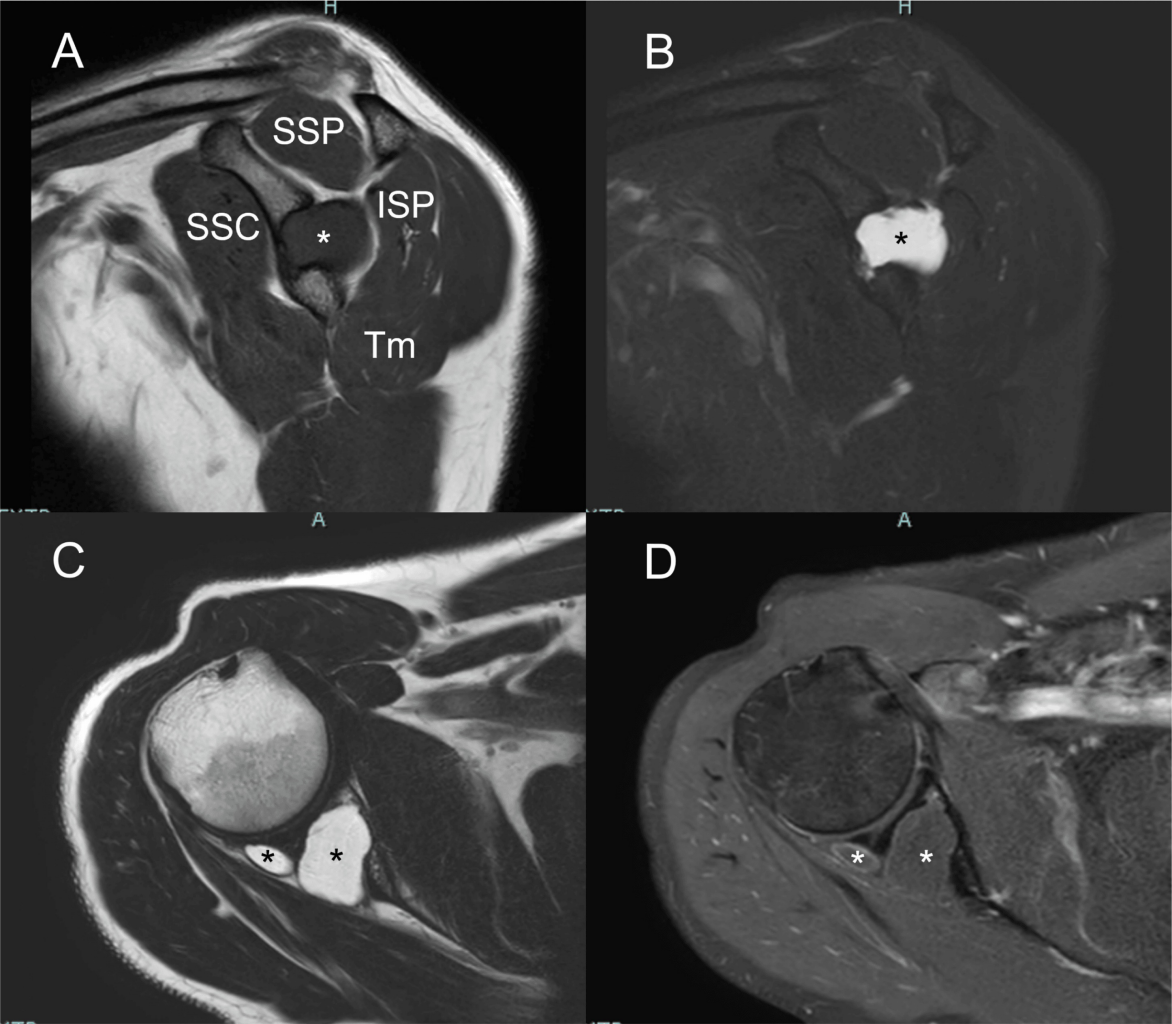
Preoperative right shoulder magnetic resonance imaging The preoperative (A) T1- and (B) T2-weighted fat suppression images in the sagittal plane and (C) T2-weighted and (D) gadolinium-enhanced T1-weighted fat suppression images in the axial plane demonstrating an intraosseous cystic lesion with enhanced margins extended to the spinoglenoid notch. *, partly multi-nodule cystic lesion; SSP, supraspinatus; ISP, infraspinatus; Tm, teres minor; SSC, subscapularis
